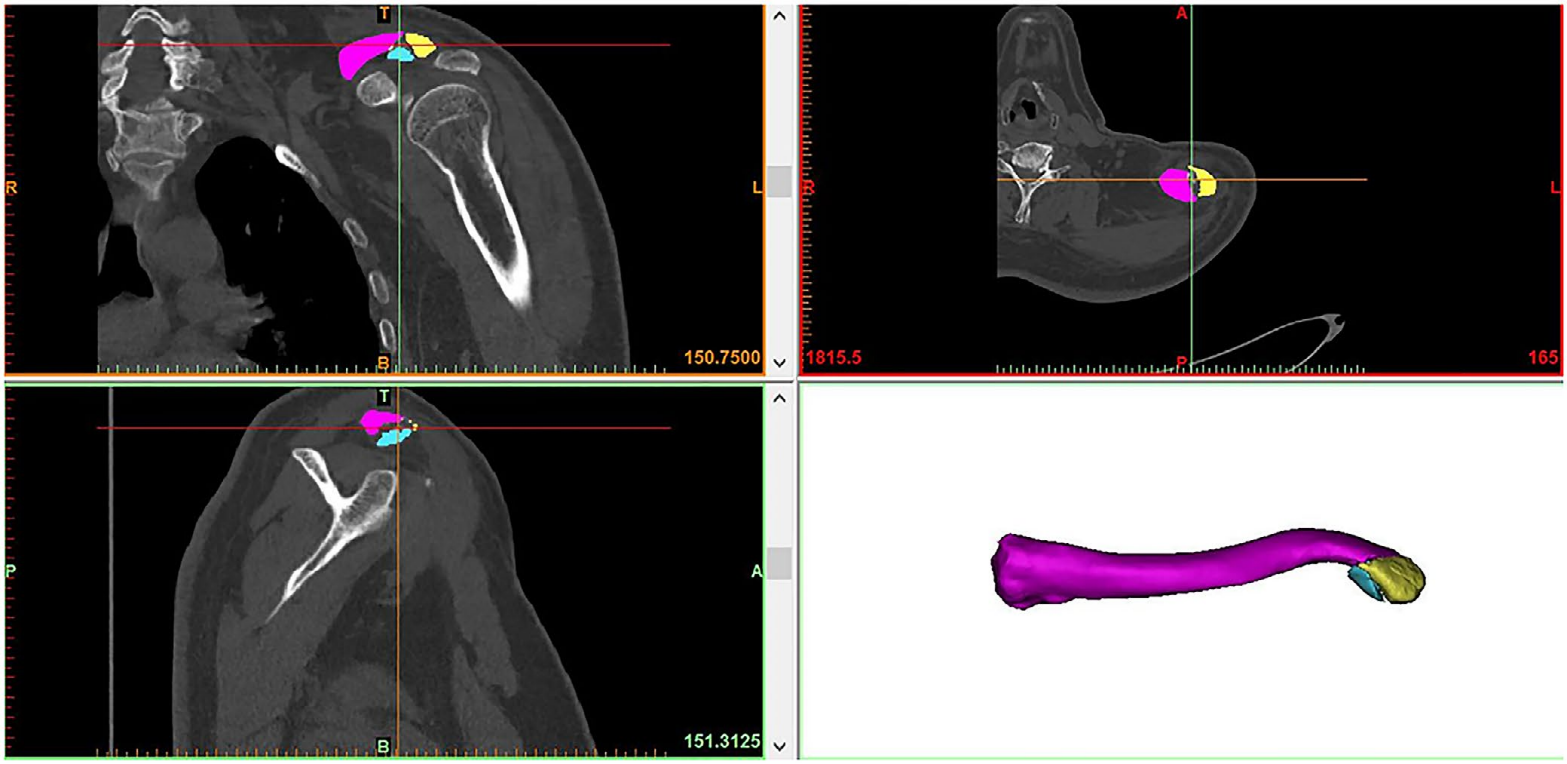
Fracture reconstruction map. The CT imaging data are imported into FirePlus 3D Medical Design software, and the fracture model is reconstructed by aligning specific landmarks: Conus nodus, acromial joint surface, acromial end, and sternal end with appropriate rotation and identification of fracture fragments.
